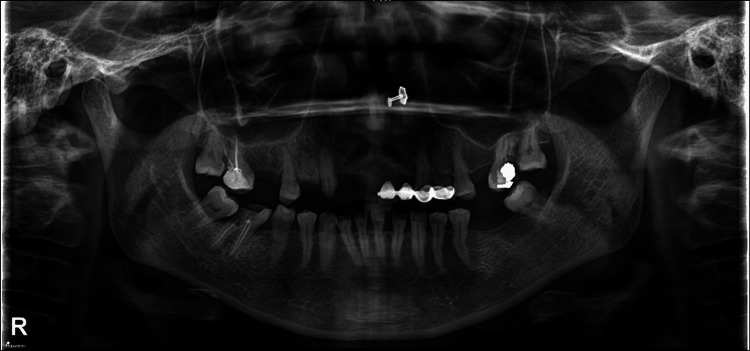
Pre-operative orthopantomograph (Case 1)
